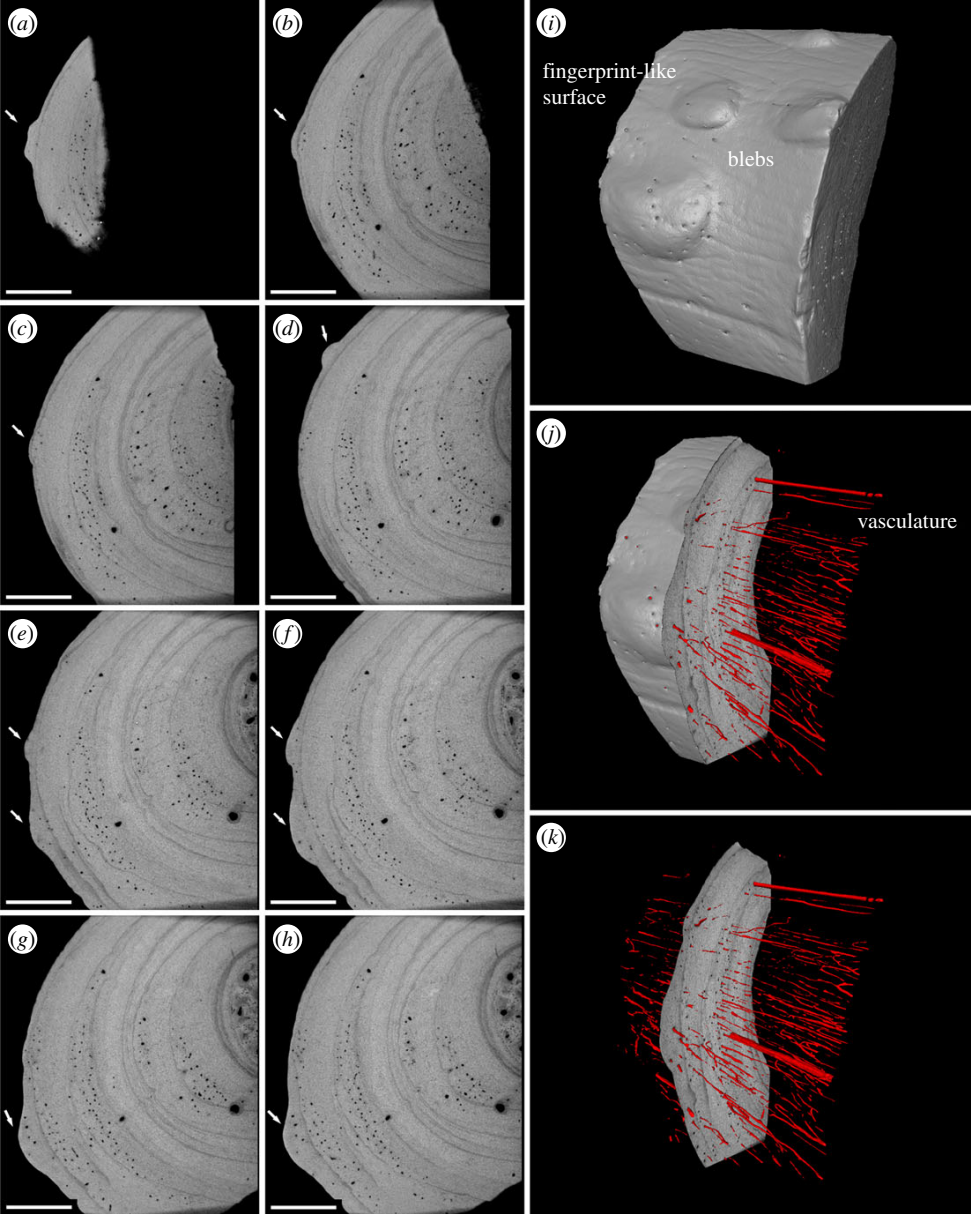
X-ray microtomographic images and three-dimensional reconstruction of the dorsal rib fragment of ‘*Proneusticosaurus*’ *silesiacus* holotype, MG UWr. 4438s. (*a*–*h*) XMT virtual sections (distal towards proximal) of the rib fragment showing superficial blebs (arrows). Note the increased vasculature within the blebs, and the bleb-like shape of older zones. Scale bar equals 1 mm. (*i*–*k*) Three-dimensional visualizations of several blebs on the rib surface as well as bone vasculature in the cropped fragment of cortical bone. Note the presence of a fingerprint-like system of superficial vascular grooves (*i*). (*i*–*k*) Not to scale. Ventral (visceral) towards the left.
